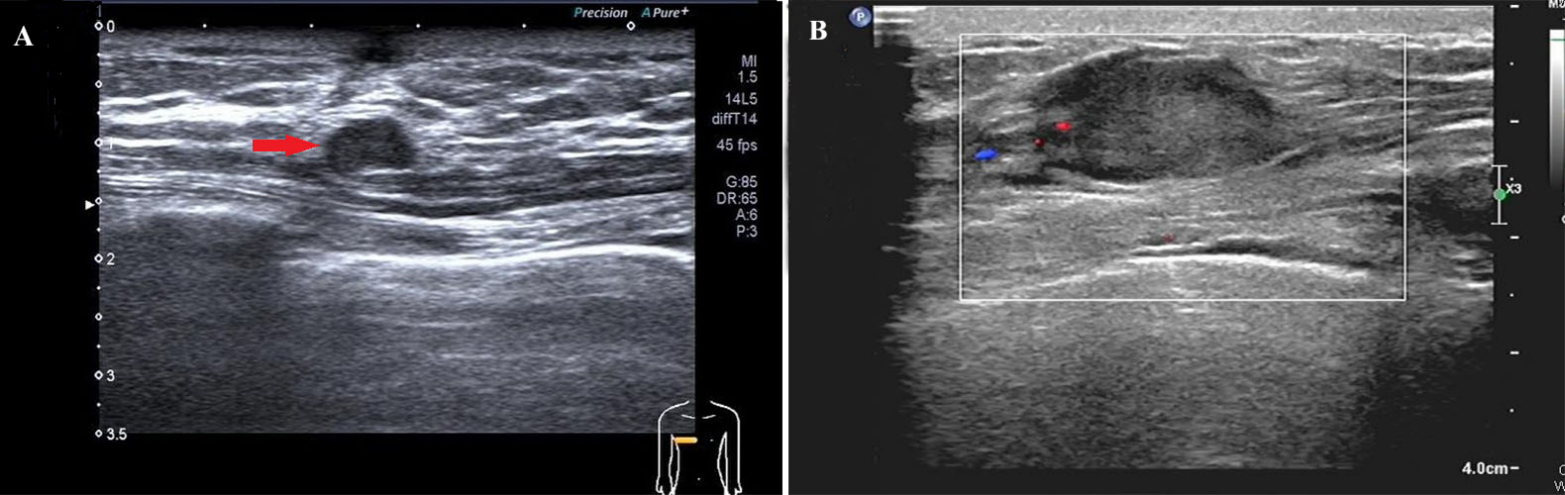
Ultrasound of the breast mass in the breast region. (A) Right breast ultrasound (April 2023): A small nodule in the right breast, measuring 0.88 cm × 0.54 cm (indicated by the red arrow). (B) Right breast ultrasound (December 2023): A nodule in the right breast, measuring 2.32 cm × 2.35 cm (within the rectangular frame).
